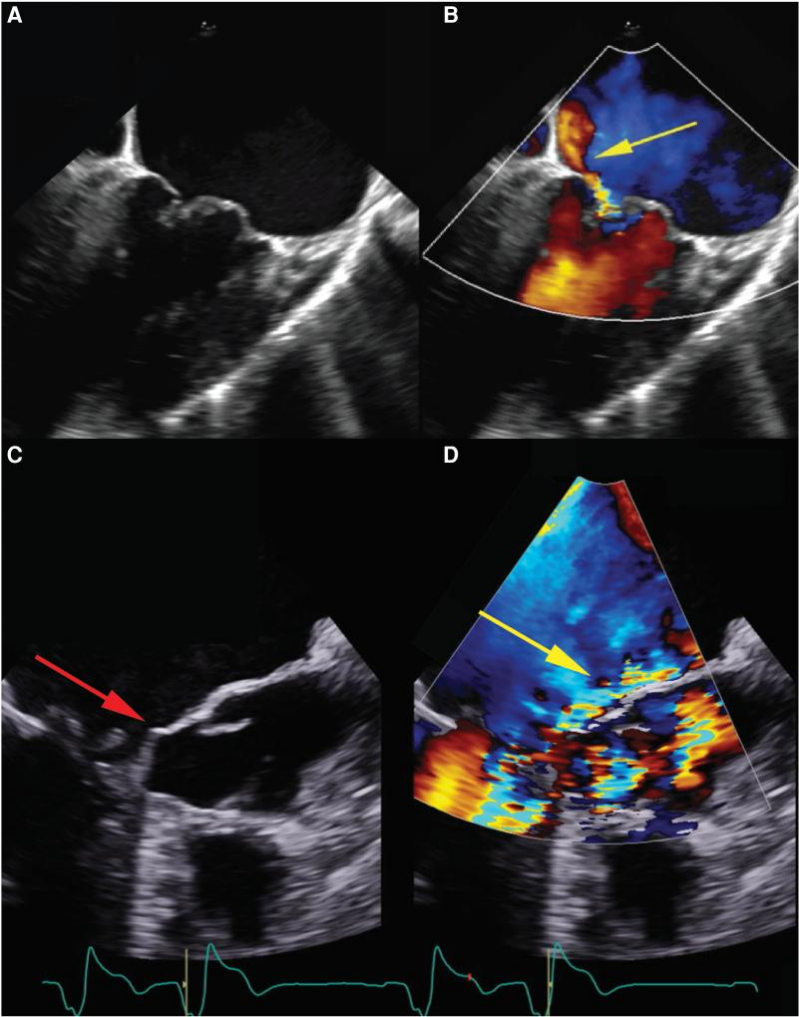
Trans-oesophageal echocardiography, mid-oesophageal mitral bi-commissural view demonstrating mitral valve P2/3 posterior leaflet prolapse (*A*) as the cause of anteriorly directed SAM (arrow) (*B*). SAM of the mitral valve (arrow) (*C*) and anteriorly directed mitral regurgitation (arrow) (*D*).
